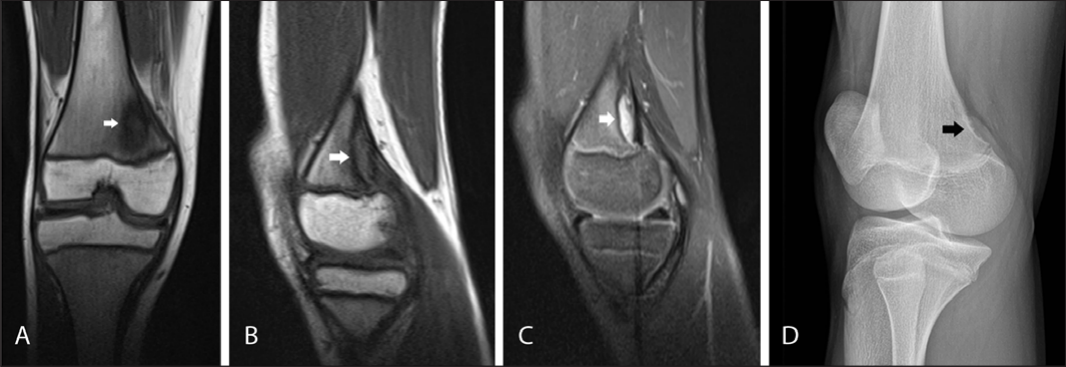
Cortical avulsive irregularity. Coronal (A) and sagittal (B) T1-WI show a well delineated hypointense defect at the posteromedial femur above the level of the growth plate (arrow). On sagittal FS T2-WI (C), the lesion is of high signal but has a hypointense border (arrow). There is no associated soft tissue mass. Oblique plain film (D) shows cortical avulsive irregularity of the knee in another patient. The lesion is typically located at the posteromedial condyle of the femur, above the growth plate of the distal femur. The radiolucent defect is often surrounded by a rim of sclerosis (arrow).
